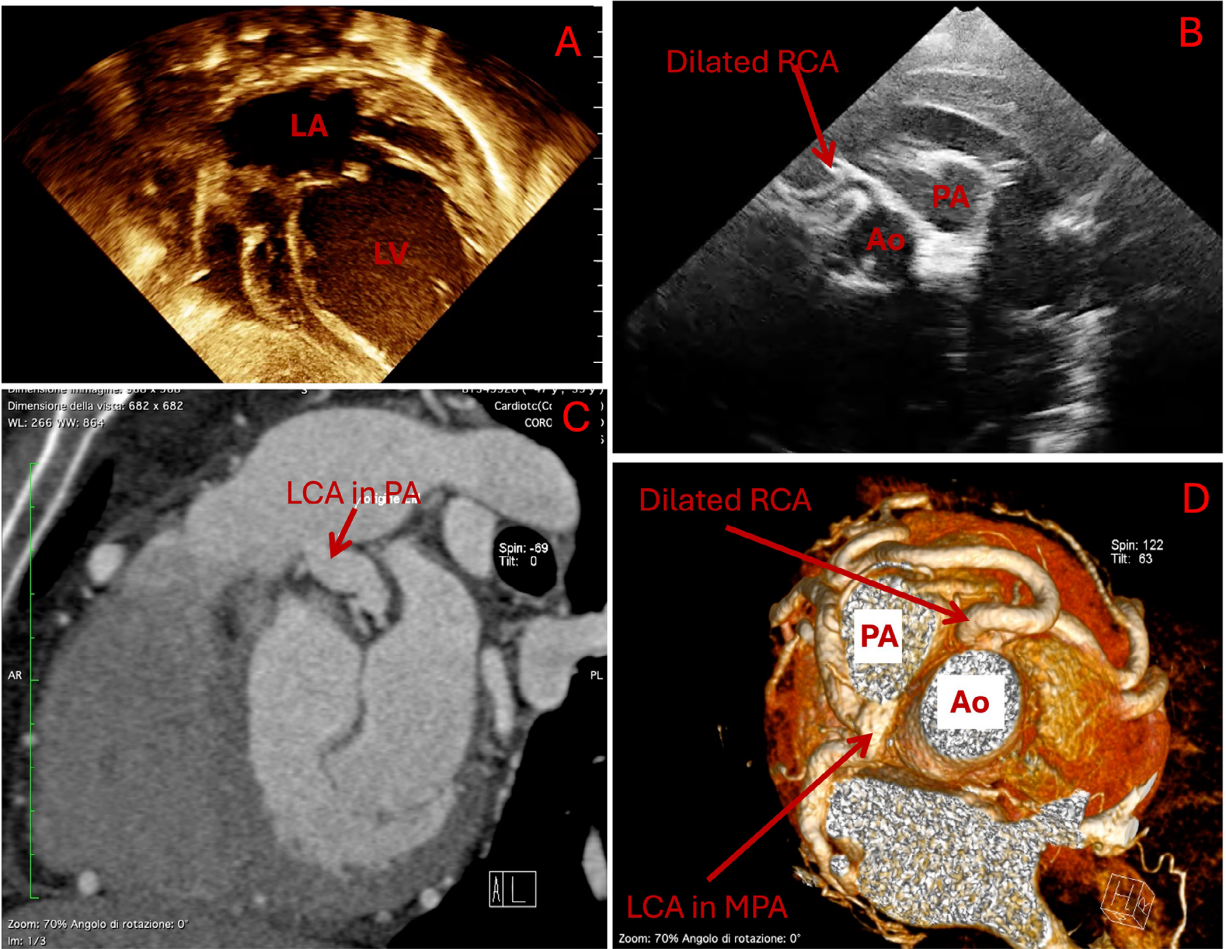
Anomalous origin of the left main coronary artery from the pulmonary artery. (A) Left ventricle was severely dilated. (B) Right coronary artery was dilated and well visible from a short‐axis view. (C) CT scan showed drainage of left coronary artery into pulmonary artery (inverted flow across the vessel). (D) 3D reconstruction of the heart. Both the coronaries were dilated. Several collateral vessels can be seen. LCA drainage in main pulmonary artery can be easily appreciated. Ao, aorta; LA, left atrium; LCA, left coronary artery; LV, left ventricle; PA, pulmonary artery; RCA, right coronary artery.
